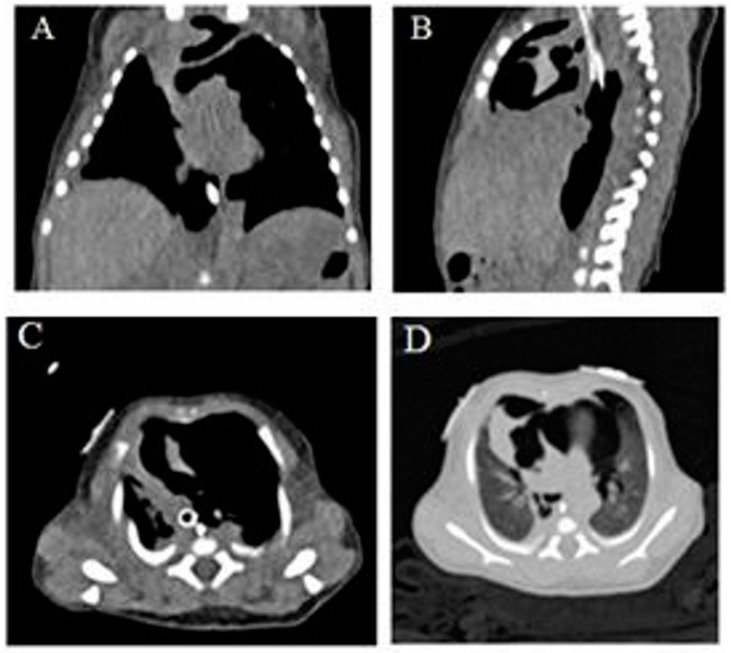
Computed tomograph of chest without contrast showing large PM (A–D).
